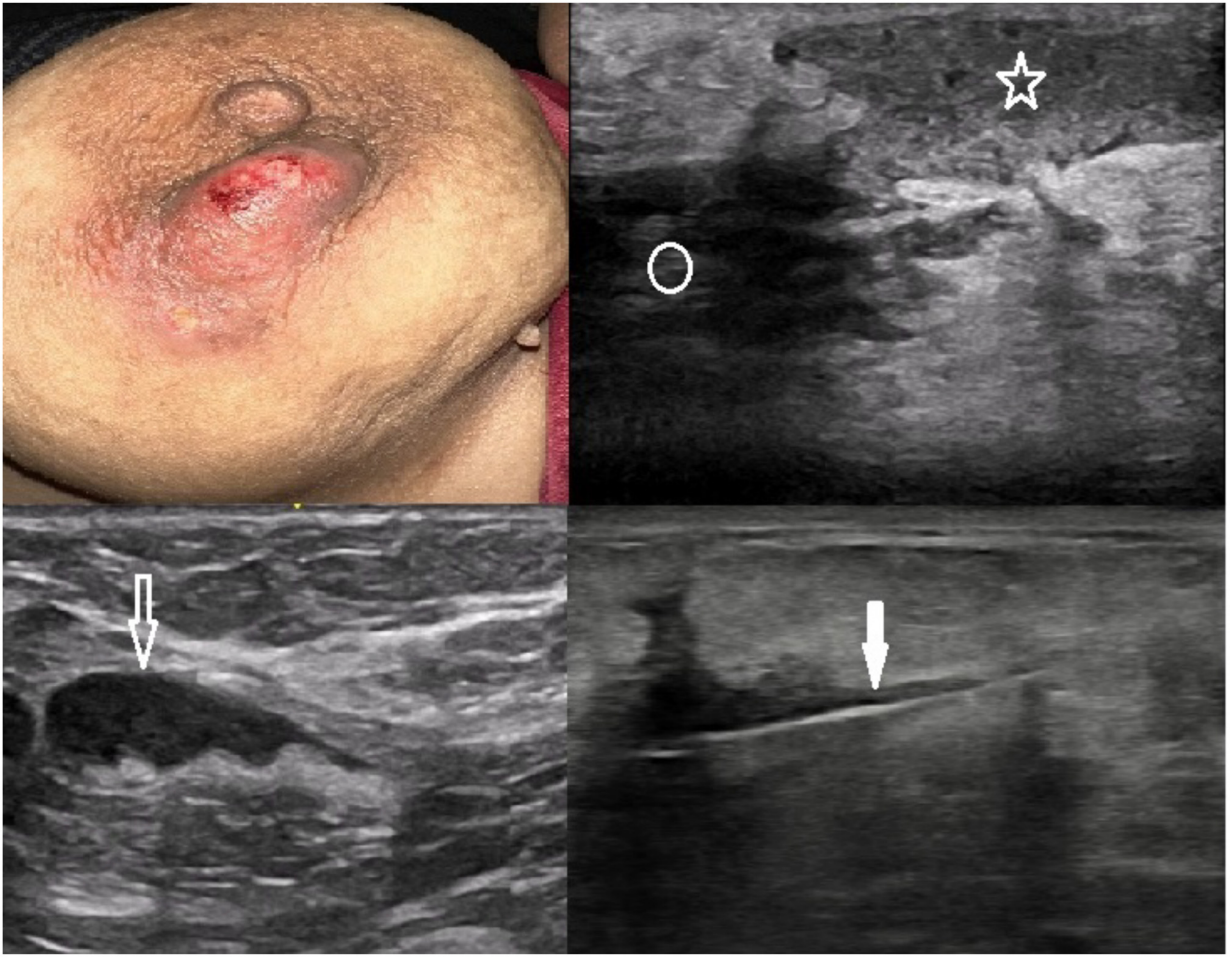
Clinical and ultrasonographic (USG) findings in a 34-year-old woman diagnosed with idiopathic granulomatous mastitis. The patient presented with pain, swelling, and erythema in the areolar region of the left breast. USG examination revealed an irregular hypoechoic lesion (indicated by a circle) within the breast tissue, a collection area (marked with a star) consistent with a superficial abscess beneath the skin, and a lymph node demonstrating asymmetric cortical thickening (denoted by an open arrow) in the axilla. Additionally, the image shows a USG-guided tru-cut biopsy being performed on an irregular hypoechoic lesion (indicated by a closed arrow) in the same patient.
